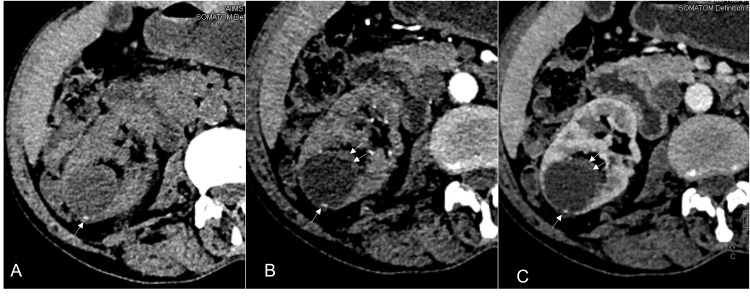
A 50-year-old male presented with right lumbar pain and a right renal cyst detected on ultrasound. Axial NCCT (A), CECT corticomedullary phase (B), and nephrographic phase (C) images show a cyst with homogenous water density in the right kidney with an eccentric calcification speck along its posterior wall (single arrow). The anterior cyst wall is minimally thickened (<3 mm) and shows enhancement (double arrows). This is categorized as a Bosniak IIF cyst. NCCT: non-contrast-enhanced CT; CECT: contrast-enhanced CT
